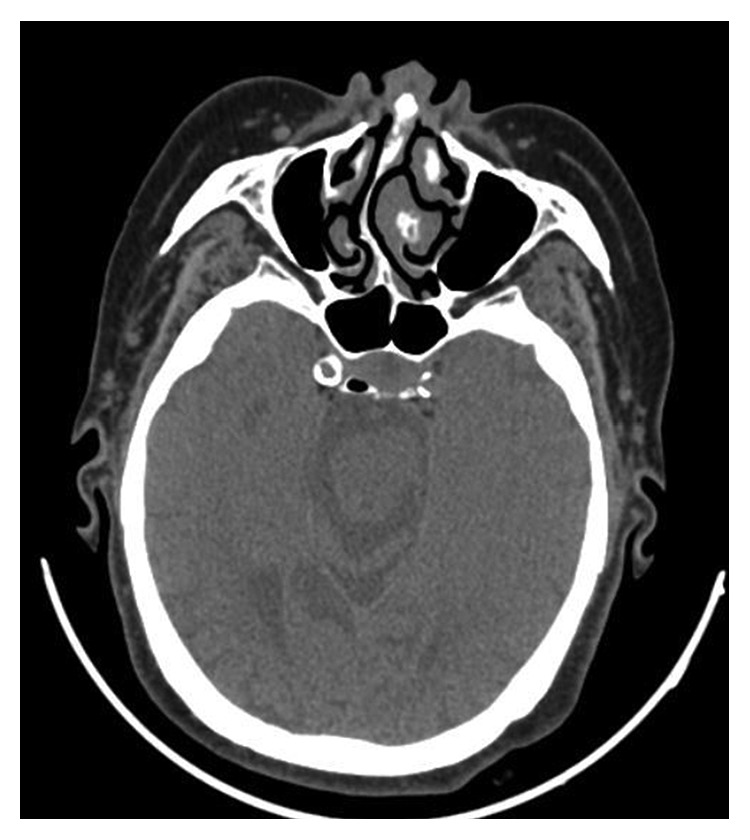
Axial section of temporal bone CT. Bilaterally, the maxillary sinuses have a natural appearance. The nasal cavity has no additional pathology except deviation of the septum.
